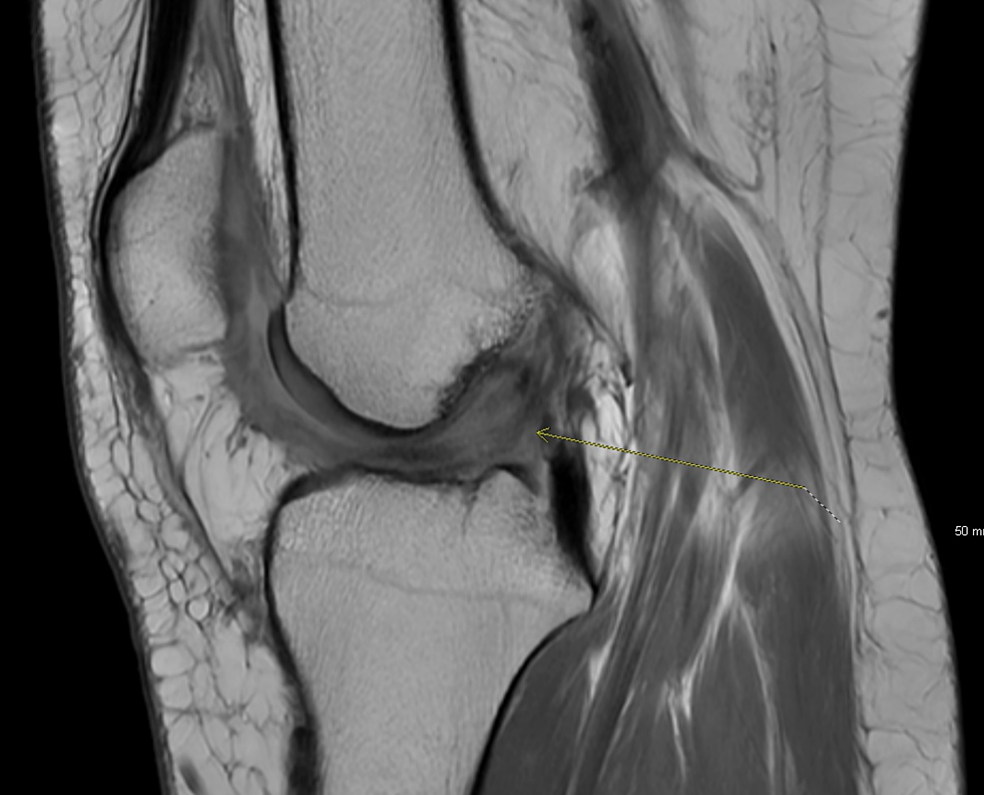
Sagittal MRI of the patient's right knee three months post-operatively demonstrating expected post-surgical changes following BEAR procedure. BEAR: bridge-enhanced ACL repair; ACL: anterior cruciate ligament
